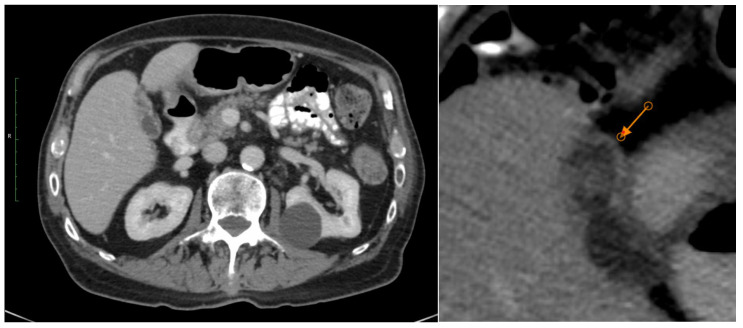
CT of abdomen with IV contrast: documentation of cholecystitis without evidence of stones or biliary tree obstruction.
